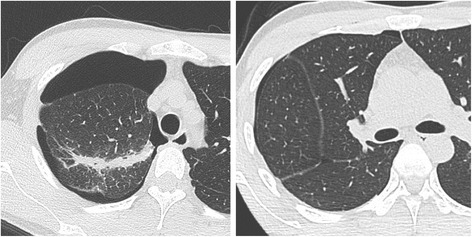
a Before the second procedure, chest computed tomography did not show any newly developed bullae near the suture line. (b) No blebs were apparent on the surface of S^6^
